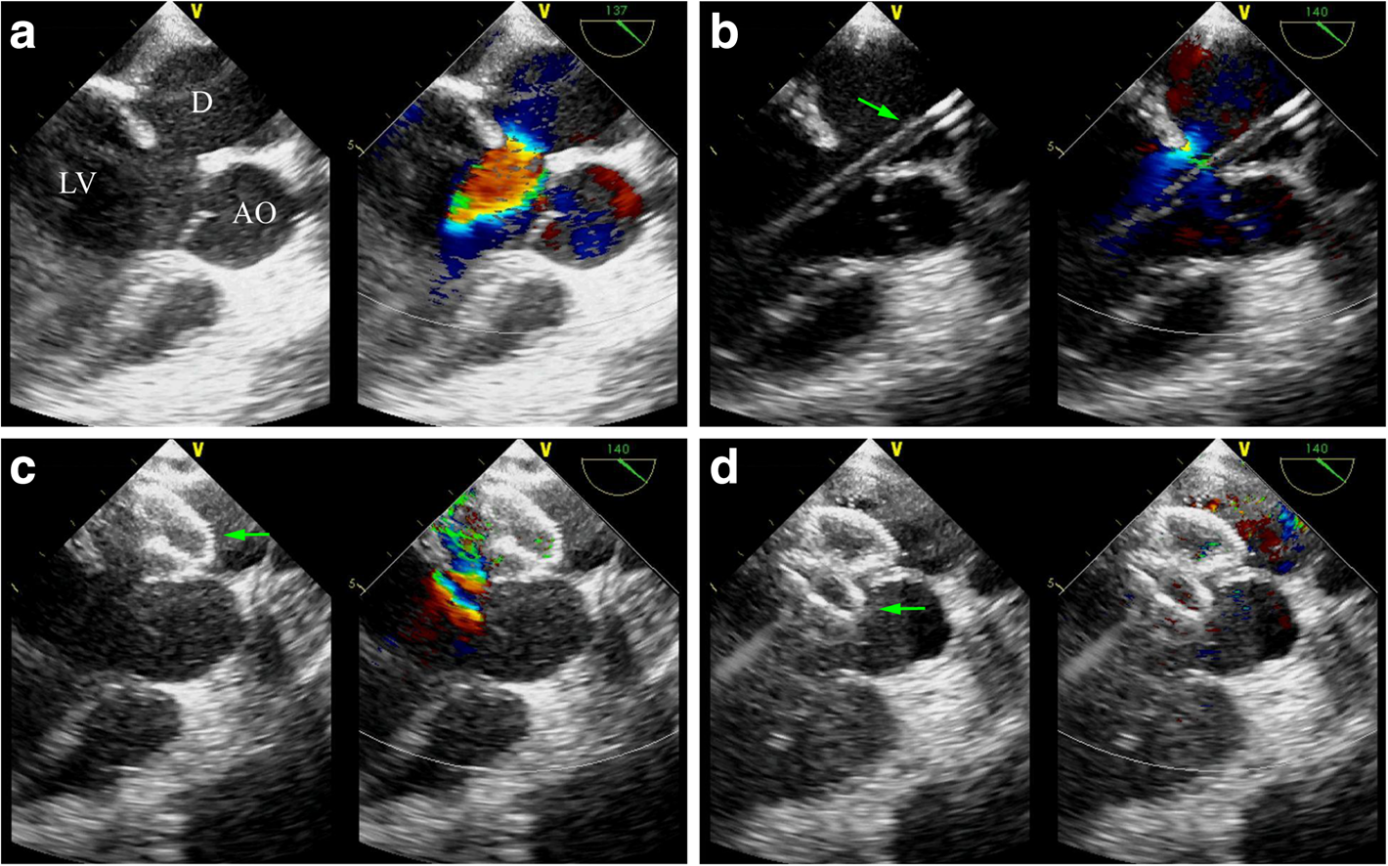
Surgical approach of transapical transcatheter device closure of relapse *left* ventricular diverticulum under transesophageal echocardiography (TEE) guidance. LV, *left* ventricle; AO, aorta; D, *left* ventricular diverticulum
